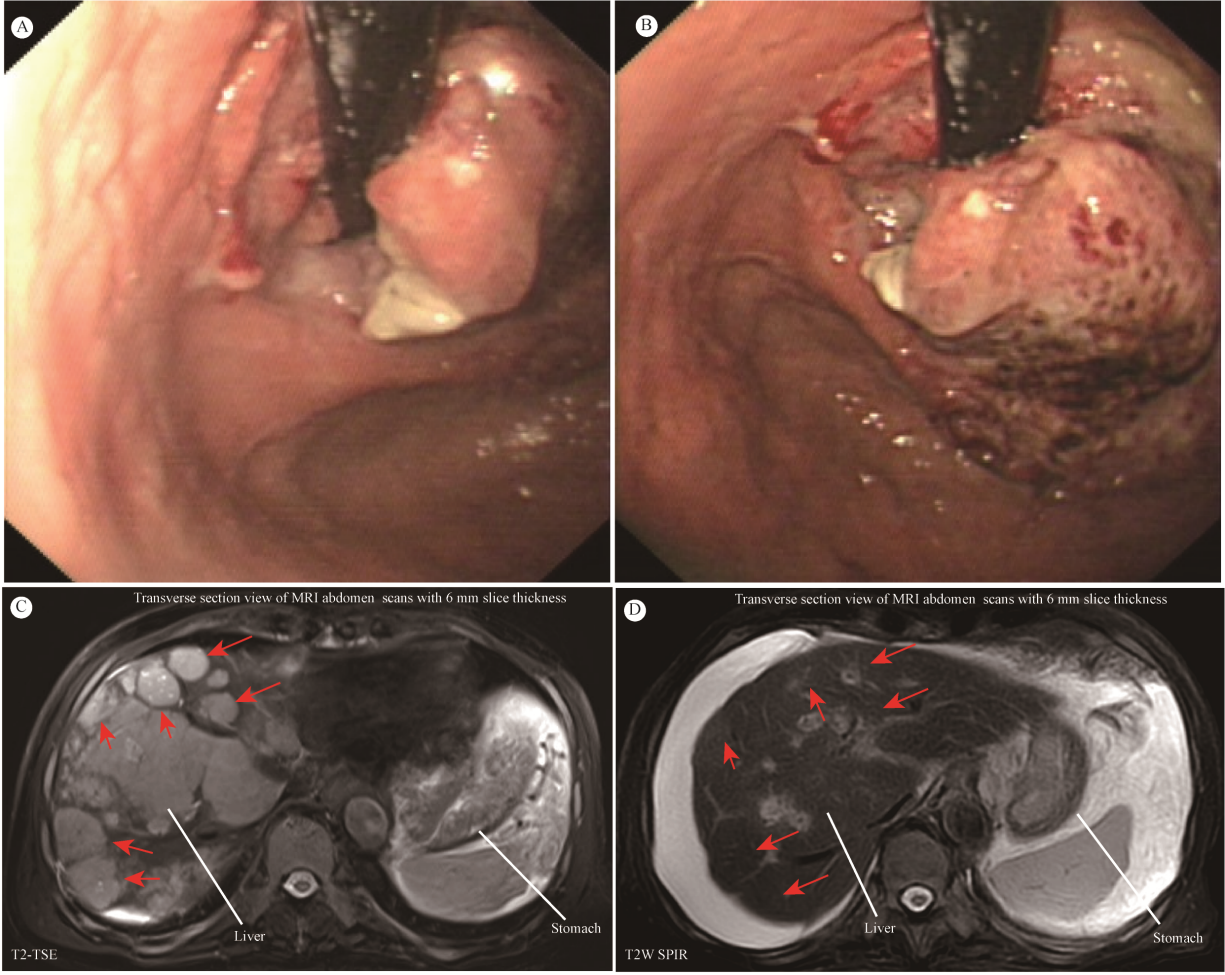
(A, B) Giant space-occupying lesion at the gastric fundus. (C, D) MRI of the abdomen. Contrast-enhanced MRI of the abdomen showed that the size and number of multiple liver metastatic tumor lesions have notably shrunk after more than four months of monotherapy with nivolumab (red arrow).
